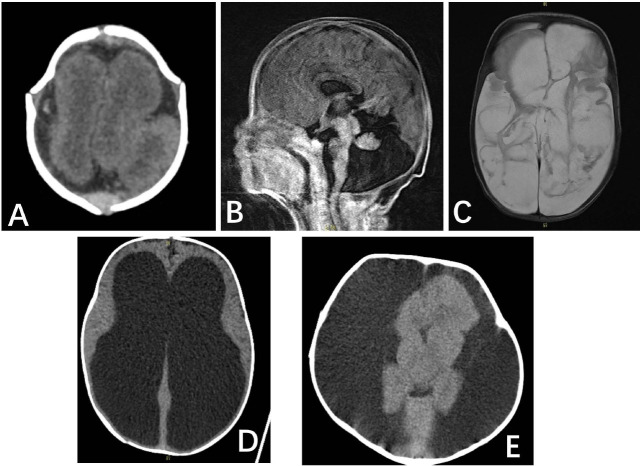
A One-month-old newborn. CT showed the lissencephaly malformation; B, one-month-old newborn, MRI showed Dandy-walker malformation with corpus callosum deficiency, he died three months after birth; C, two-month-old newborn, T2WI showed cystic changes in the bilateral cerebral hemisphere; D, two-months-old newborn, CT indicated severe hydrocephalus with thinning of cerebral cortex who died 1 month after birth; E, 10-months-old infant, CT showed most of hemisphere was absent with massive effusion under the skull who died one year later.
